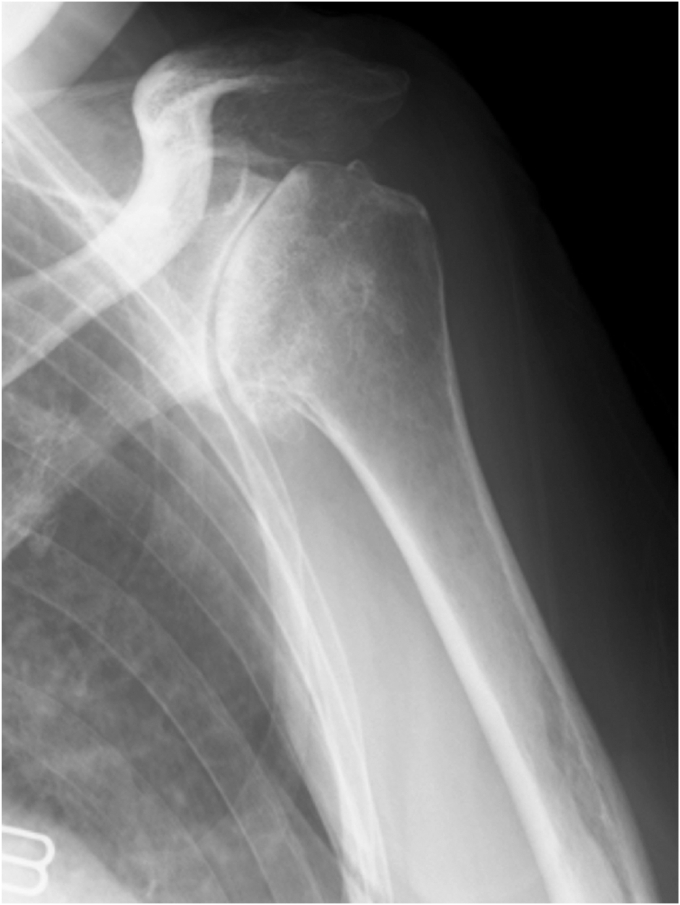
Preoperative radiographs of the left shoulder showed glenohumeral joint space narrowing, subchondral sclerosis, and osteophytes on the inferior humeral head.
